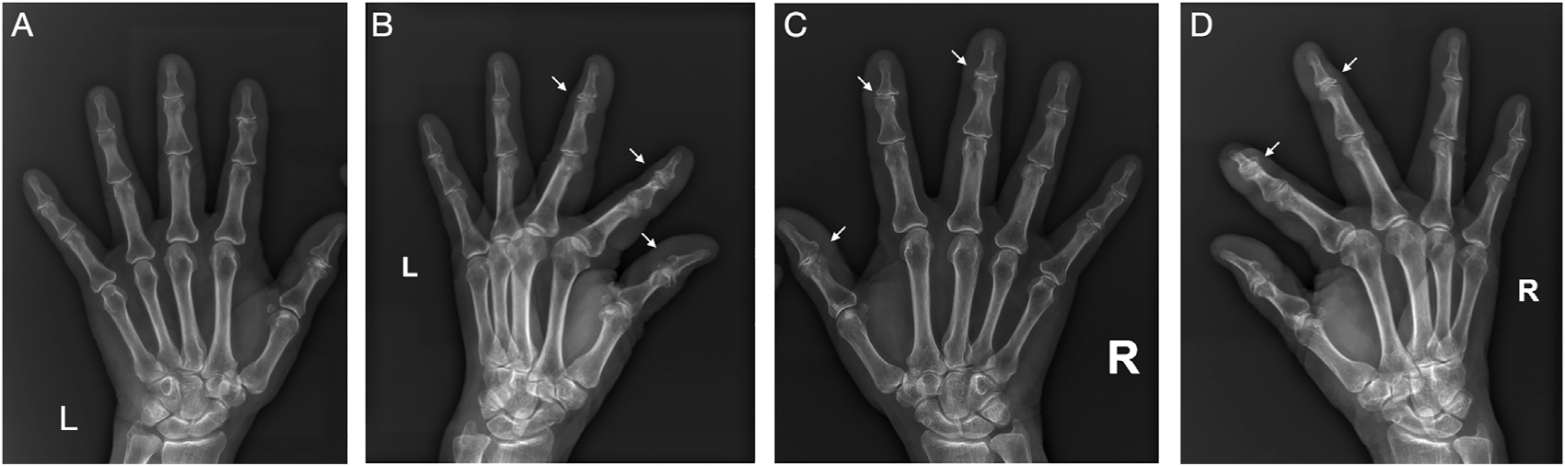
Typical X-ray films of OA in hand. This is the X-ray films of both hands of a 71-year-old obese woman (BMI 28.0). Significant manifestations of OA (narrowing of joint space, osteophyte formation, and subchondral bone sclerosis) can be seen in these non-weight-bearing joints. (A) Frontal view of left hand; (B) Lateral view of left hand; (C) Frontal view of right hand; (D) Lateral view of right hand.
